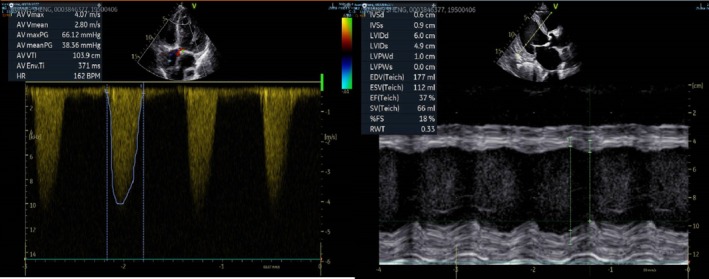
Pre‐procedure transthoracic echocardiography. The mean transaortic gradient of 38 mmHg, the maximum transaortic gradient of 66 mmHg, the outflow tract velocity of 4.1 m/s and the left ventricular ejection fraction was 37%.
